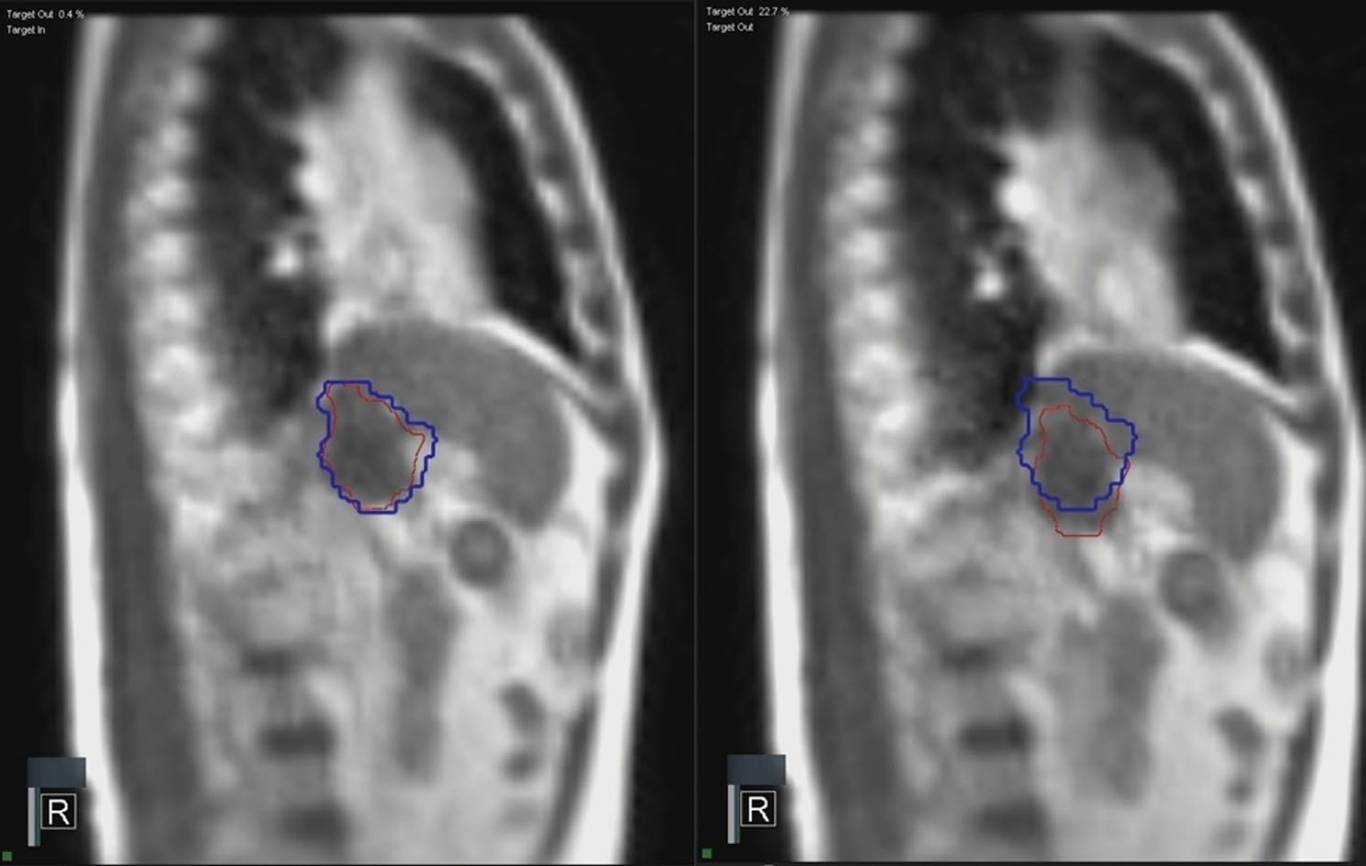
Real time MRI-guided tumor-tracking and gating radiotherapy through CINE MRI. Red line: Target (gross tumor volume of portal vein tumor thrombus), Blue line: Boundary (5 mm margin from target). During radiotherapy, the beam was automatically turned off if the target moved outside the boundary by more than 3%. MRI, magnetic resonance imaging
